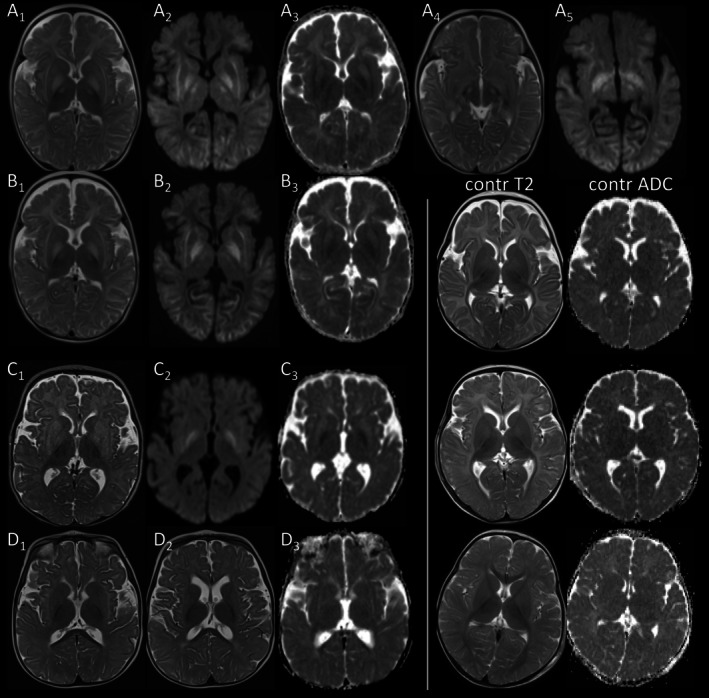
Basal ganglia changes in younger patients. (A, B) T2‐hyperintense pallidum, anterolateral thalamus, and subthalamic nucleus with restricted diffusion in p1 at 0.3 years (A) on day 2 of first acute metabolic decompensation with. Putamen and caudate nuclei are less T2‐hyperintense with facilitated diffusion. (B) Repeat MRT after 3 days (having become comatose) reveals no significant change, in particular no restricted diffusion in striatum. (C, D) T2‐hyperintense putamen and pallidum with restricted diffusion of pallidum on day 4 of first acute metabolic decompensation in p2 at 0.4 years (C). T2‐hyperintensity and restricted diffusion of pallidum have normalized by follow‐up at 0.7 years (D). There is residual, faint, mottled T2‐hyperintensity of putamen (compare with signal of age‐matched control). (T2w: A_1_–D_1_, A_4_, D_2_, DWI: A_2_, A_5_, B_2_–D_2_, ADC‐maps: A_3_–D_3_.)
